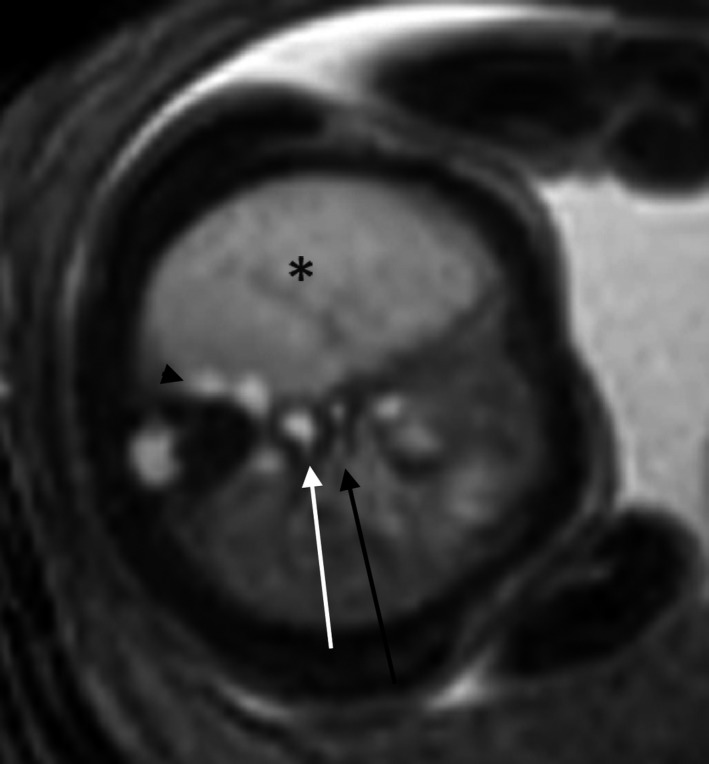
Axial SSFP MRI sequence of the fetal chest demonstrating the relationship of the carina (black arrow), foregut duplication cyst (white arrow), partially imaged cluster of small cysts which extended further inferior (black arrowhead), and the area of right midlung overinflation (asterisk)
